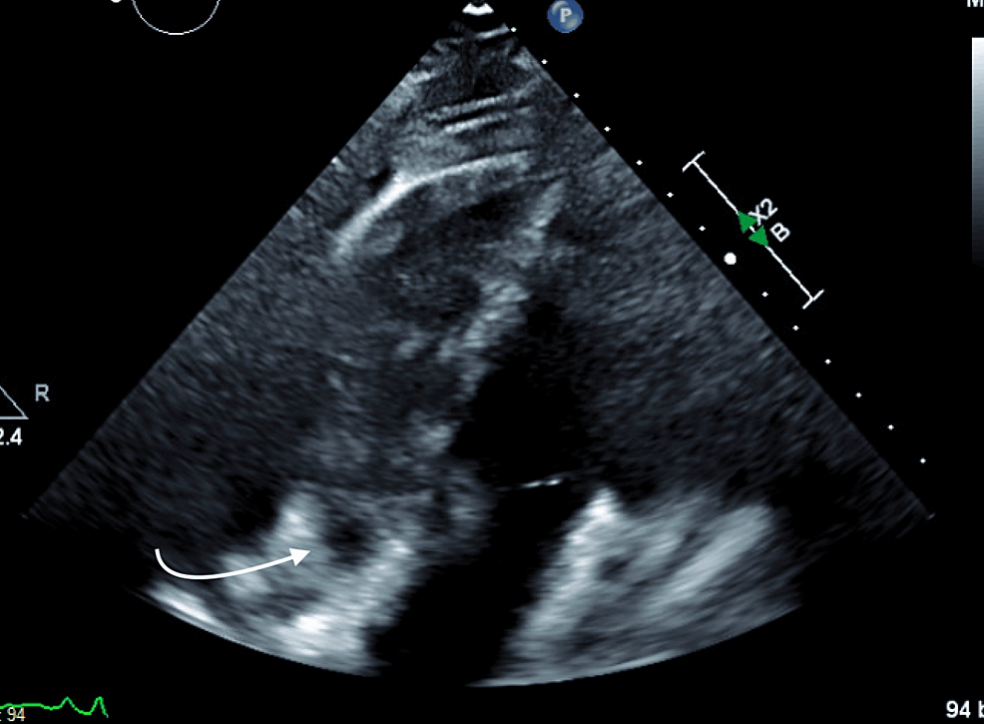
Transthoracic echocardiogram showing right atrium mass.
